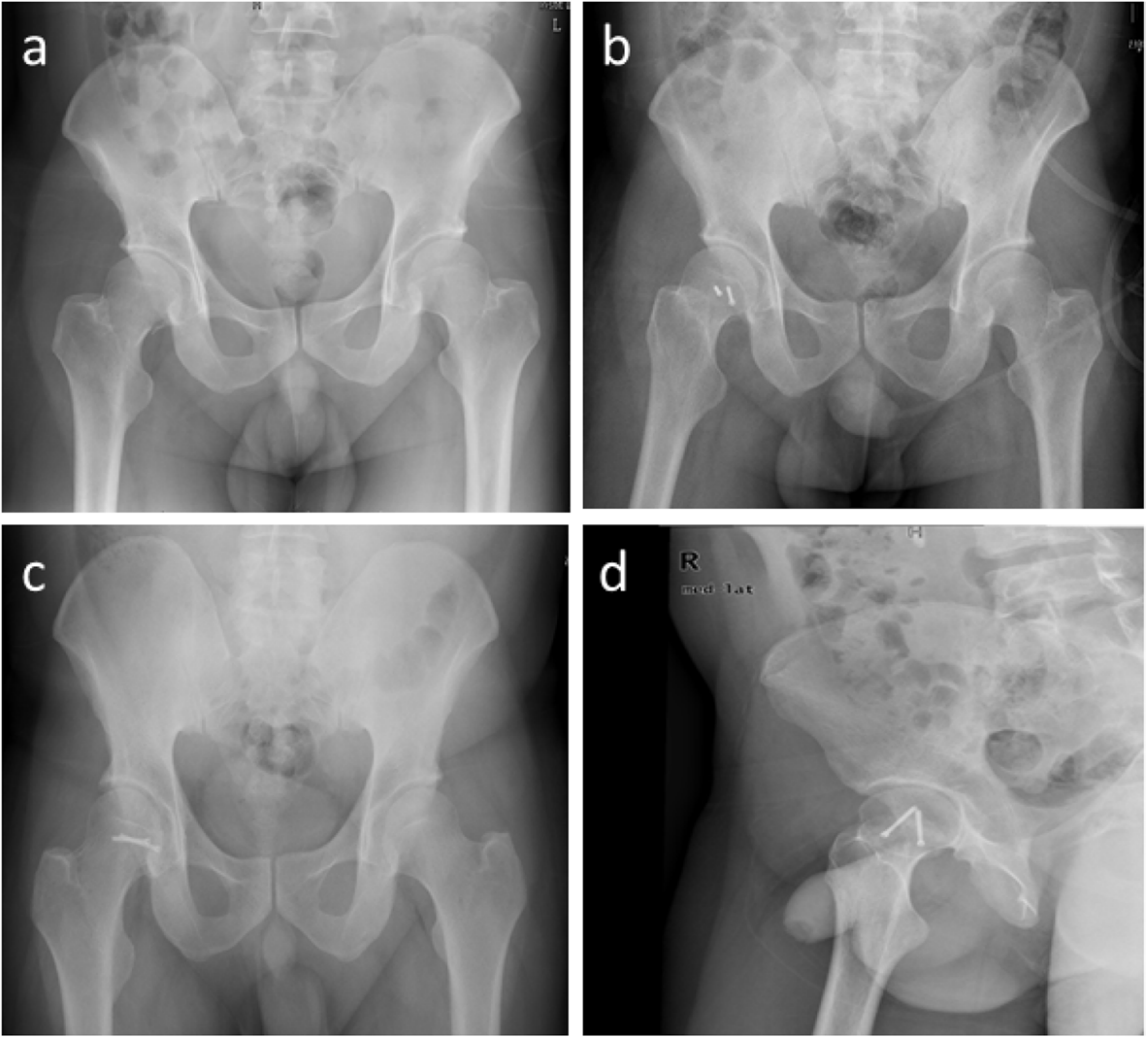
a A 39 -year-old man with Pipkin type I right femoral head fracture b Anteroposterior (AP) radiographs showing anatomical reduction of the femoral head using anterior approach under direct vision. c AP and d Lateral radiograph of the hip during 3rd-year follow-up after internal fixation
